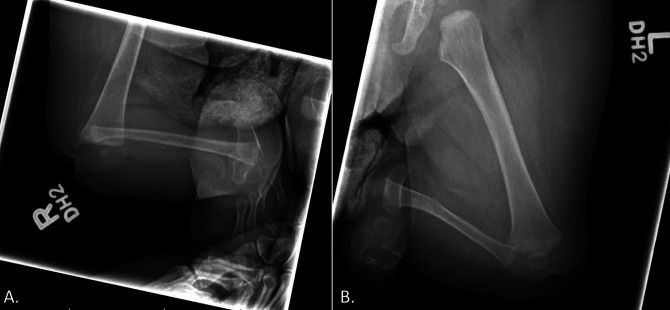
Radiographic anterior-posterior imaging when the patient was of 8 months. A, Right lower extremity demonstrating absent tibia with dislocation and severe flexion contracture of the knee and subluxation or dislocation of the ankle. B, Anterior-posterior view of the left lower extremity demonstrating absent tibia with dislocation or subluxation of the knee and dislocation of the ankle.
